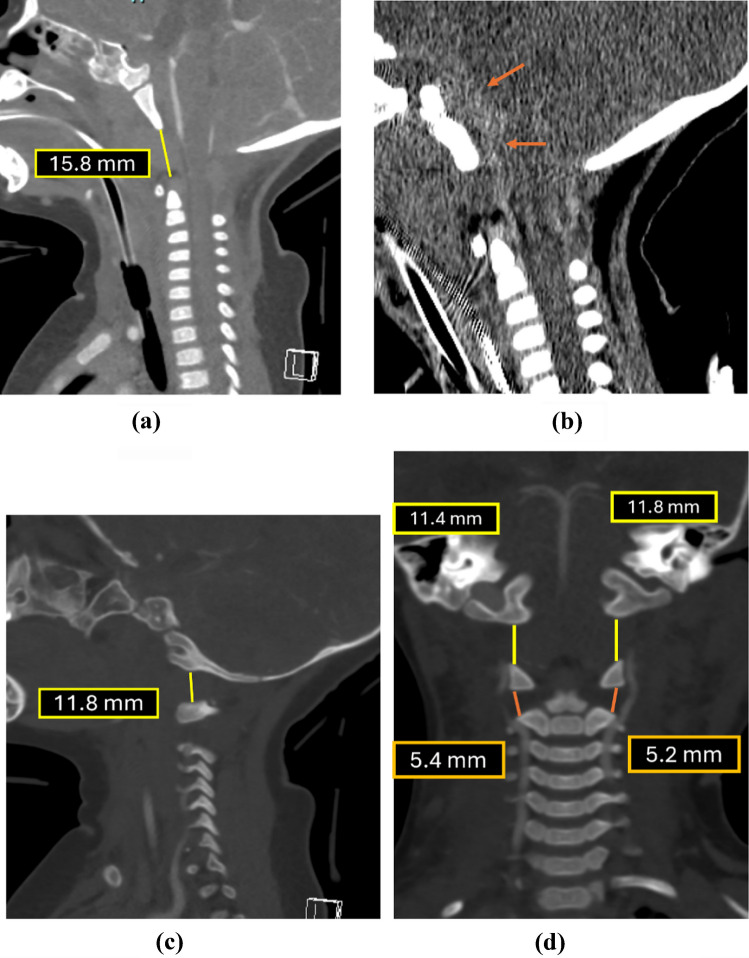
The initial CT was interpreted as normal, with no evidence of fracture. On detailed review, the following findings were noted: (a) basion–cartilaginous dens interval (BCDI) was markedly increased at * 15.8  mm* (normal upper limit for age <6 years: *5.6 mm*); (b) retroclival hyperdensity became evident after adjusting window settings (*orange arrows*); (c) parasagittal CT images demonstrated a significantly widened  occipital condyle -C1 interval with V-shaped widening of the joint space and elevated distance (normal <2.5 mm); (d) coronal CT images revealed both an increased occipital–condyle-C1 interval (normal <3.5 mm) and elevated atlanto-axial interval (normal <3.9 mm). No fractures were identified
